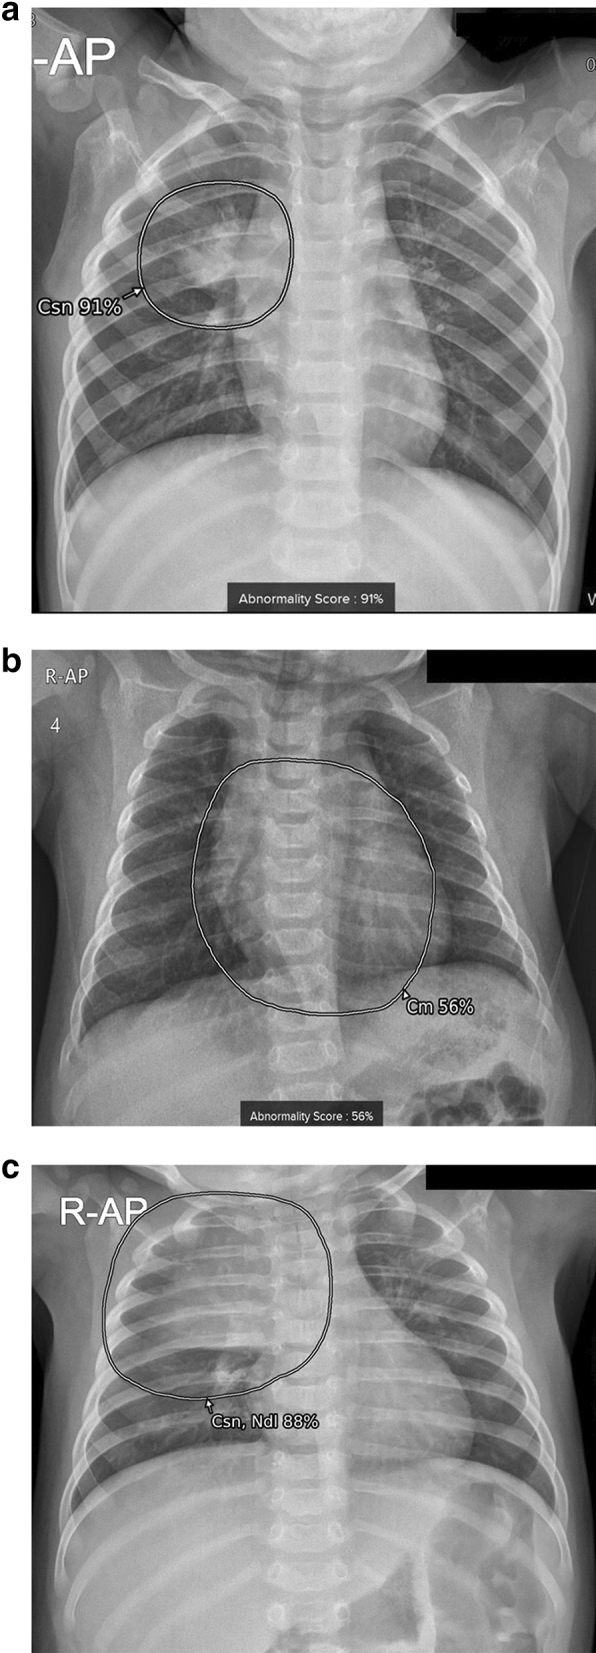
Examples of results analyzed by the AI-based lesion detection software. (a) A 17-month-old boy with pneumonia in the right upper lobe. The software detected consolidation (Csn) with an abnormality score of 91% in the right upper lobe, as marked in the grayscale map. (b) A 3-month-old boy with a cardiothoracic ratio of 50%, within normal range. The software detected cardiomegaly (Cm) with an abnormality score of 56% on the anteroposterior chest radiograph. (c) A 4-month-old girl without remarkable findings on the chest radiograph. The software detected normal thymus as consolidation (Csn) and nodule (Ndl) with an abnormality score of 88%.
